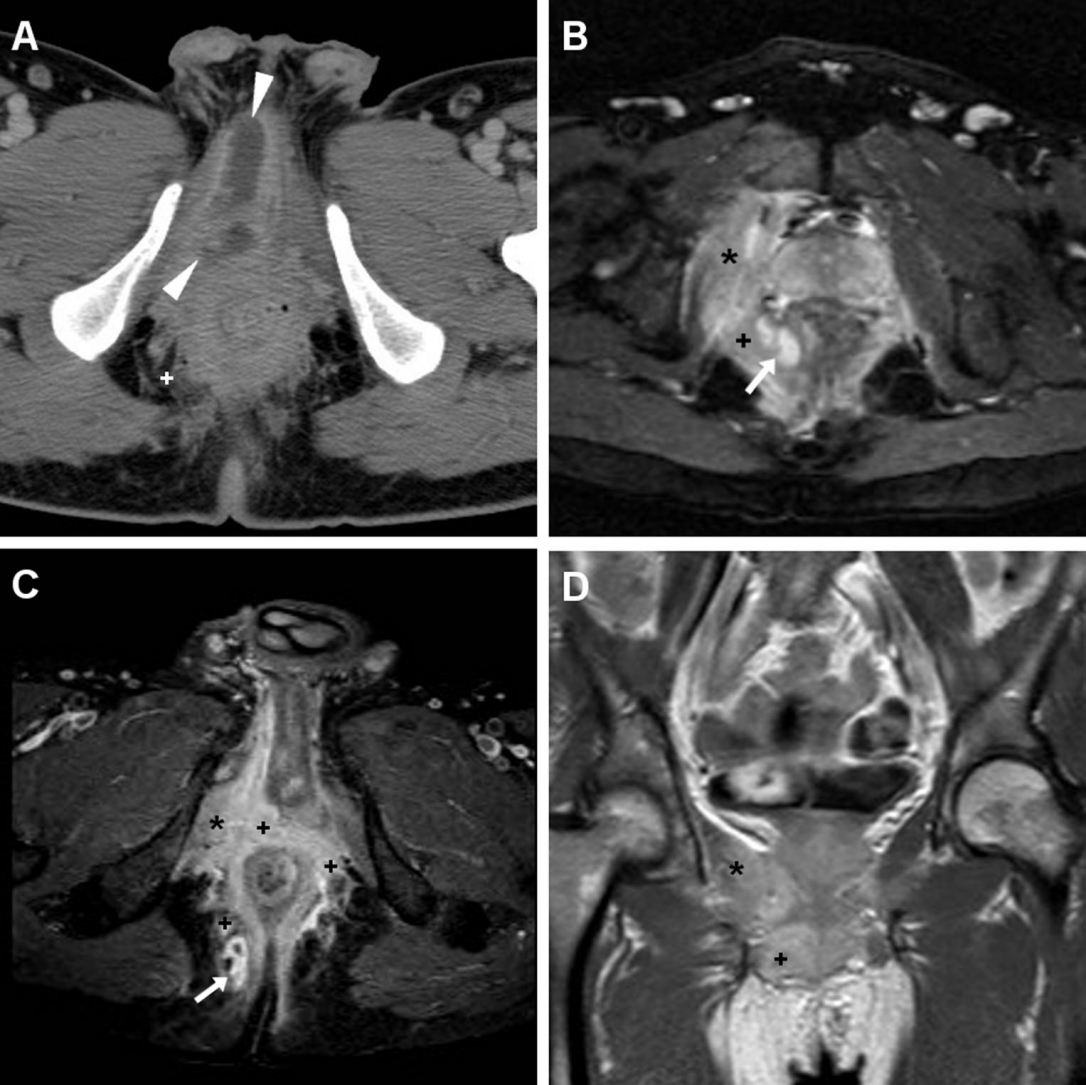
Extensive cryptogenic perianal inflammation in a 56-year old diabetic man with fever. Axial post-contrast CT image (a) revealed perineal abscess (*arrowheads*) very similar to that depicted in Fig. 15. Additional MRI including axial STIR (b), post-gadolinium axial fat-suppressed (c) and coronal (d) T1-weighted images showed extensive inflammatory signal abnormalities and hyperenhancement (+) surrounding the anus, involving the right sphincter complex and obturator internus (*) muscles, and extending to the ischioanal fossa. Tiny abscess collections (*arrows* in b and c) were present. Topography of infection, sparing of prostate and corpora cavernosa, and clinical examination were inconsistent with complicated UTI
